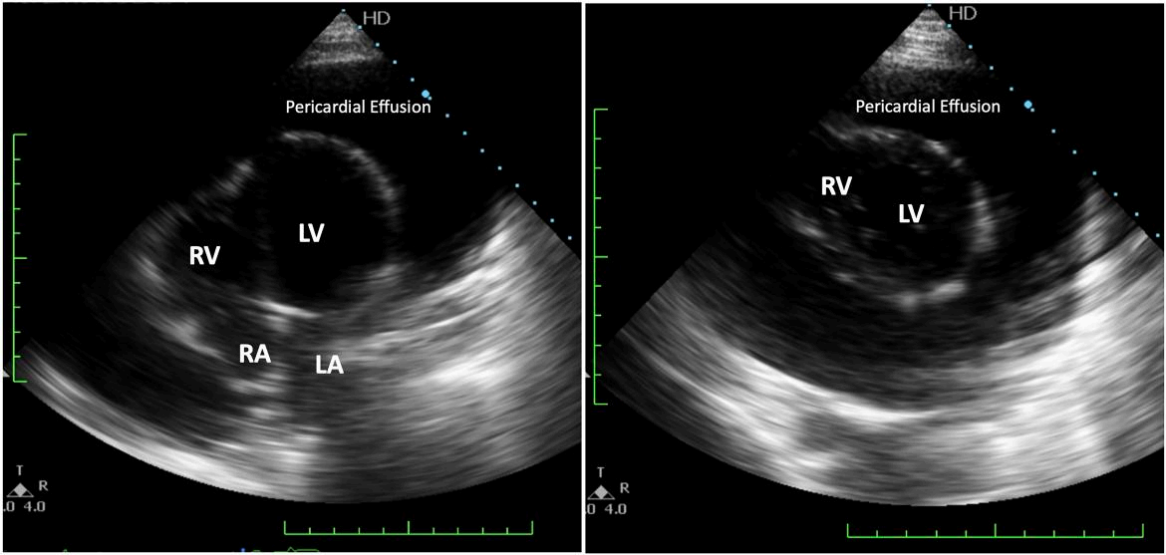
Echocardiogram from the initial emergency presentation, featuring views from the apical four-chamber and short-axis, displaying extensive pericardial fluid.
